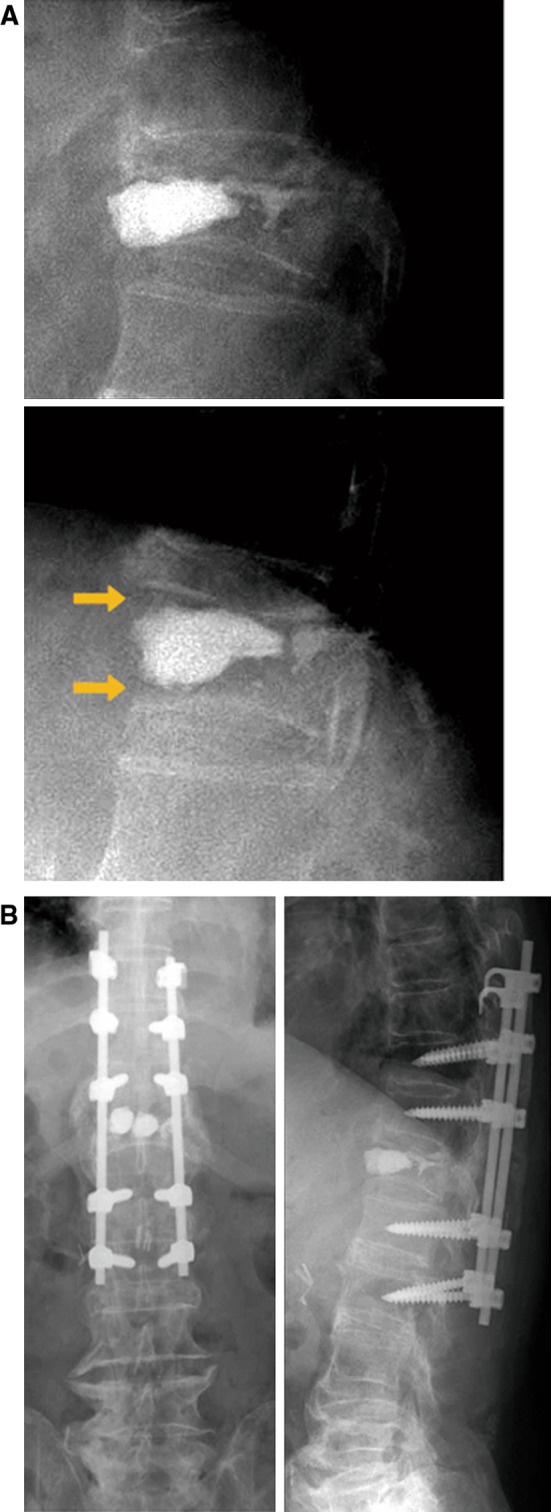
(Case 2) a Lateral spine flexion–extension radiographs demonstrated instability, and PMMA did not adhere to the endplate of the treated vertebrae. b Surgical intervention was performed by pedicle screw and hook fixation from Th10 to L2
